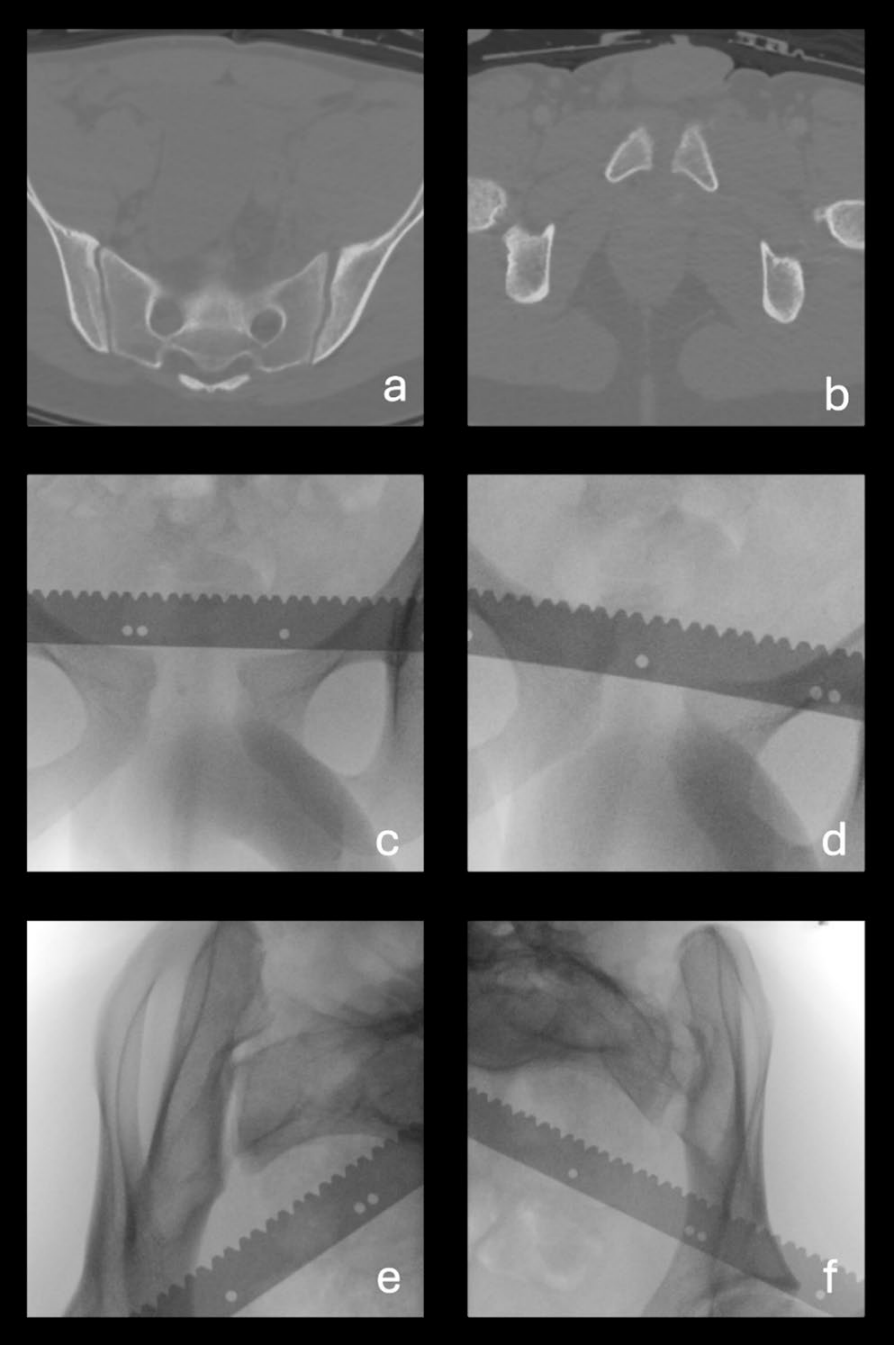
Case 18 is an example of when the classification changes from anterior-posterior compression 2 (APC2) to anterior-posterior compression 3 (APC3), where observing the sacroiliac (SI) joint allowed us to detect diastasis in both SI joints, instead of assuming that there is only diastasis in the left SI, as seen on the CT scans. (a) axial CT image of sacrum with a pelvic binder on, demonstrating diastasis in the anterior part of the left SI joint but no apparent diastasis in the posterior part of the SI joint (b) axial CT image of pubic symphysis, showing minimal symphyseal diastasis (c) Anterior-posterior (AP) examination under anaesthesia (EUA) image of the pubic symphysis in the external rotation stress maneuver showing 3.0 cm of symphyseal diastasis (d) AP EUA image of the pubic symphysis in the push/pull stress maneuver showing 1.5 cm of rotational instability (e) Obturator inlet EUA image of right SI in the external rotation stress maneuver, demonstrating anterior SI diastasis (f) Obturator inlet EUA image of left SI in the external rotation stress maneuver, demonstrating complete SI diastasis
